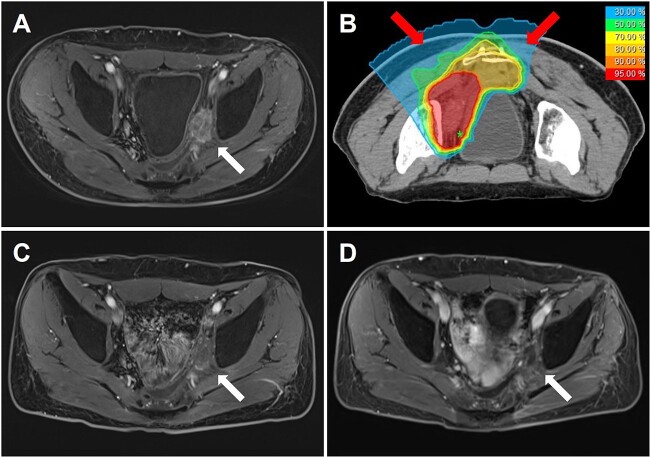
A 41-year-old male patient with pelvic side-wall recurrence of rectal cancer treated by carbon ion re-irradiation. (A) Baseline MRI with contrast enhancement before CIRT. (B) Dose distribution of the CIRT plan using 2 oblique posterior beams, with a prescription D_RBE_ of 75.6 Gy in 21 fractions. (C) Follow-up MRI 1 month after CIRT showing a responding tumor with significantly decreased enhancement. (D) Latest MRI taken 47 months after CIRT demonstrating no evidence of tumor recurrence.
